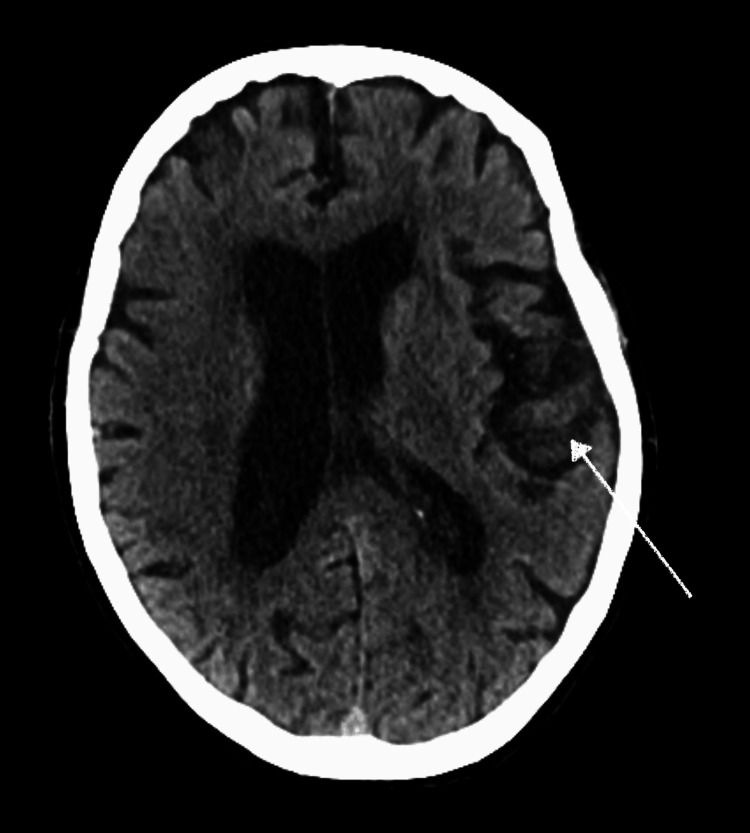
CT brain revealing focal encephalomalacia (arrow) CT: computed tomography
